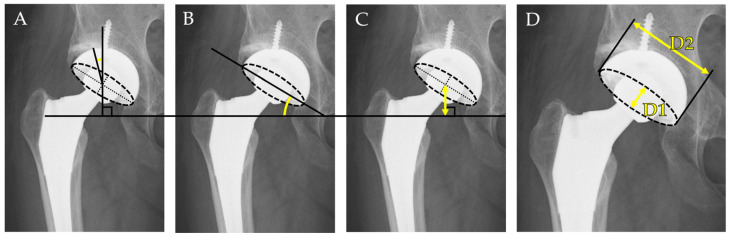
Radiographic measurement of acetabular cup positioning. (A) Center-edge (CE) angle: the angle formed between a vertical line perpendicular to the inter-teardrop line and a line connecting the center of the femoral head to the original lateral edge of the native acetabulum. (B) Inclination angle: the angle between the inter-teardrop line and the opening face of the acetabular component. (C) Cup height: the vertical distance from the femoral head center to the inter-teardrop line. (D) Radiographic anteversion: calculated using Lewinnek’s method as sin^−1^ ($\frac{D1}{D2}$), where *D1* and *D2* represent the short and long axes of the elliptical projection of the cup on anteroposterior radiographs.
